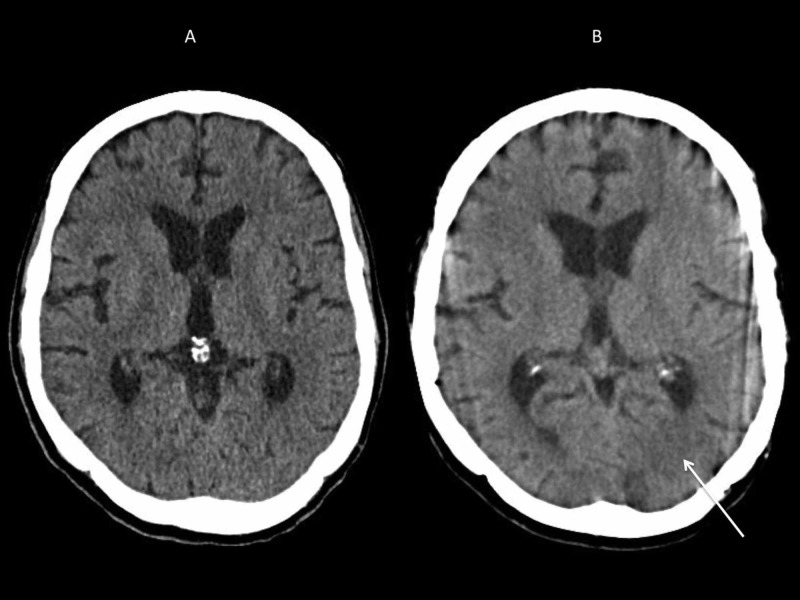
CT scan of brain on the day of admission (A) and at 48 hours after admission (B). A: CT brain performed on the day of hospitalization showed no acute intracranial pathology. B: CT brain performed at 48 hours after hospitalization showed focal abnormalities in the left parieto-occipital areas of brain (arrow).
